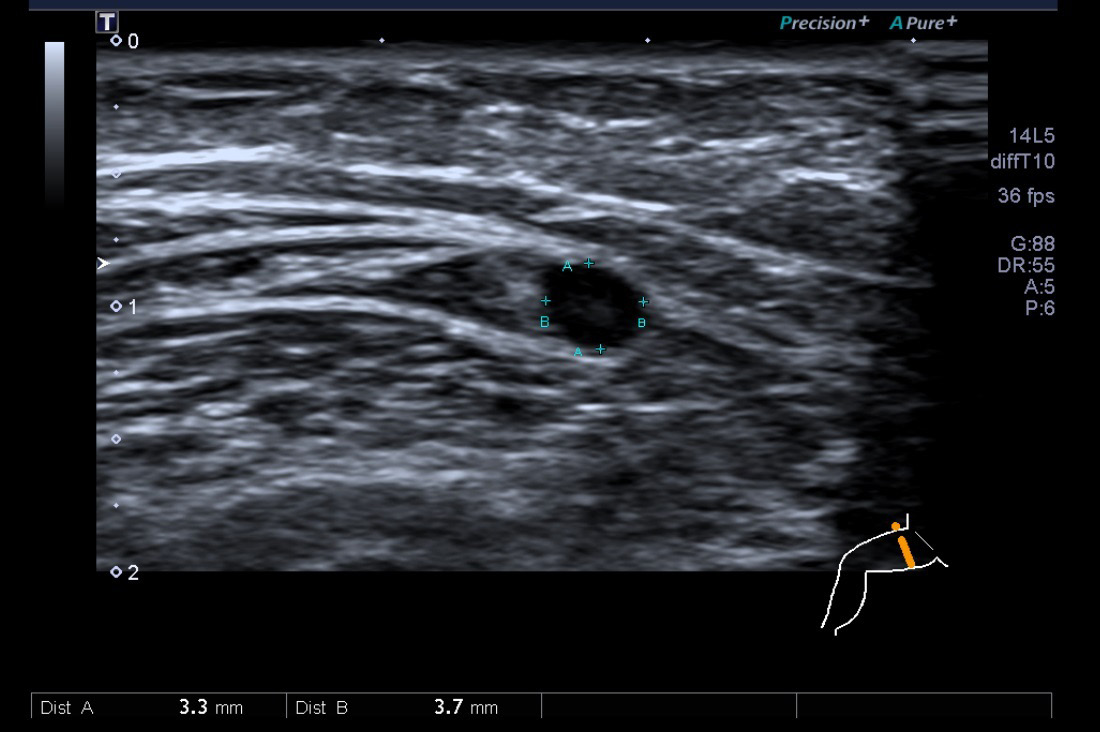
Measurement of great saphenous vein diameter with ultrasonography.
